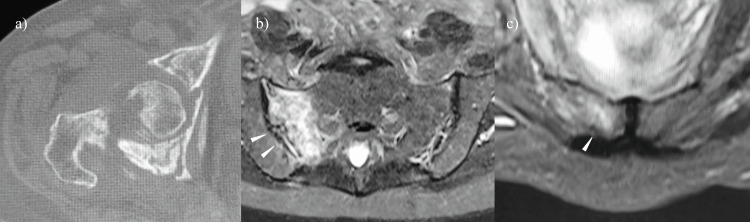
Preoperative CT and MRI of case 2 a) Preoperative CT and b, c) MRI of the T2-weighted STIR sequence The white arrow indicates b) sacral ala fracture and c) pubic ramus fracture STIR: Short Tau Inversion Recovery
